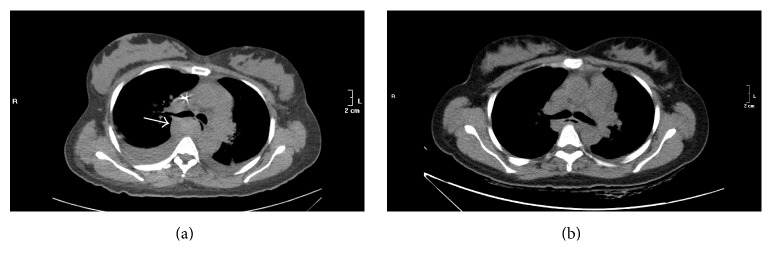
Chest CT transverse view of (a) hematoma (white arrow) displacing the esophageal lumen on 2nd day after C-section. (b) Normal esophageal anatomy and lumen patency after full recovery, 17 days after C-section.
